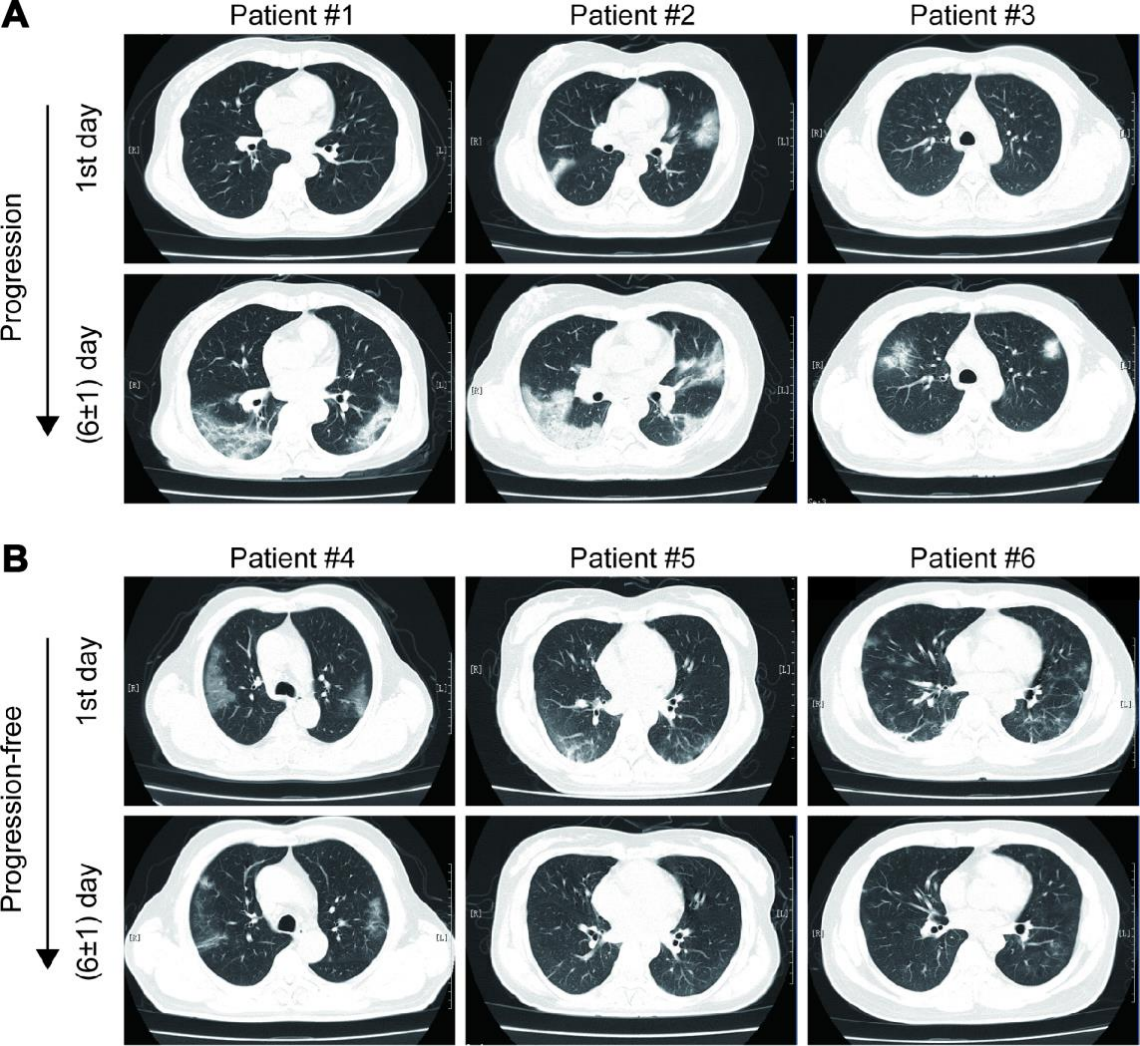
Examples of imaging progression (A) and progression-free (B) in chest CT from COVID-19 patients.
